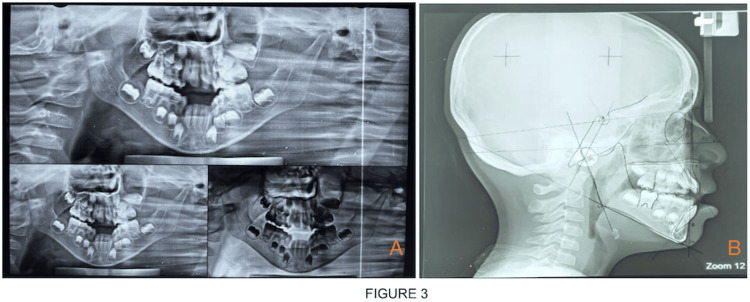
(A) Panoramic radiograph. (B) Lateral cephalometric tracing.
